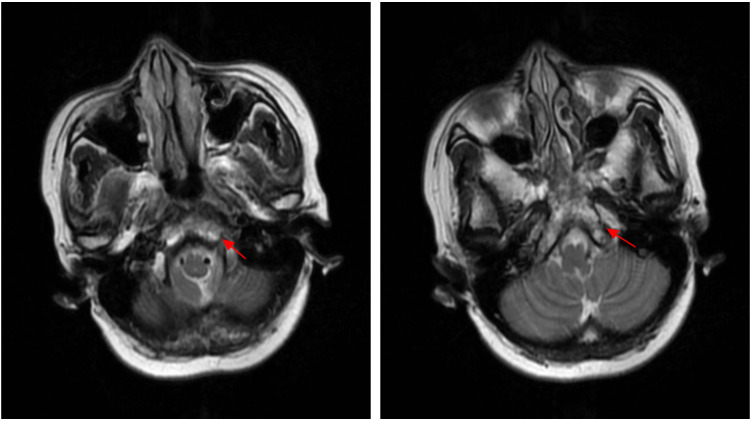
Thirty-month post-radiotherapy follow-up head MRI (T2-IDEAL sequence): Axial images demonstrate sustained regression of the clivus lesion (red arrows). The lesion size and signal intensity are reduced compared with those at one month post-radiotherapy. No evidence of local recurrence or new intracranial lesions is observed. These findings indicate durable disease control at 30 months post-treatment.
